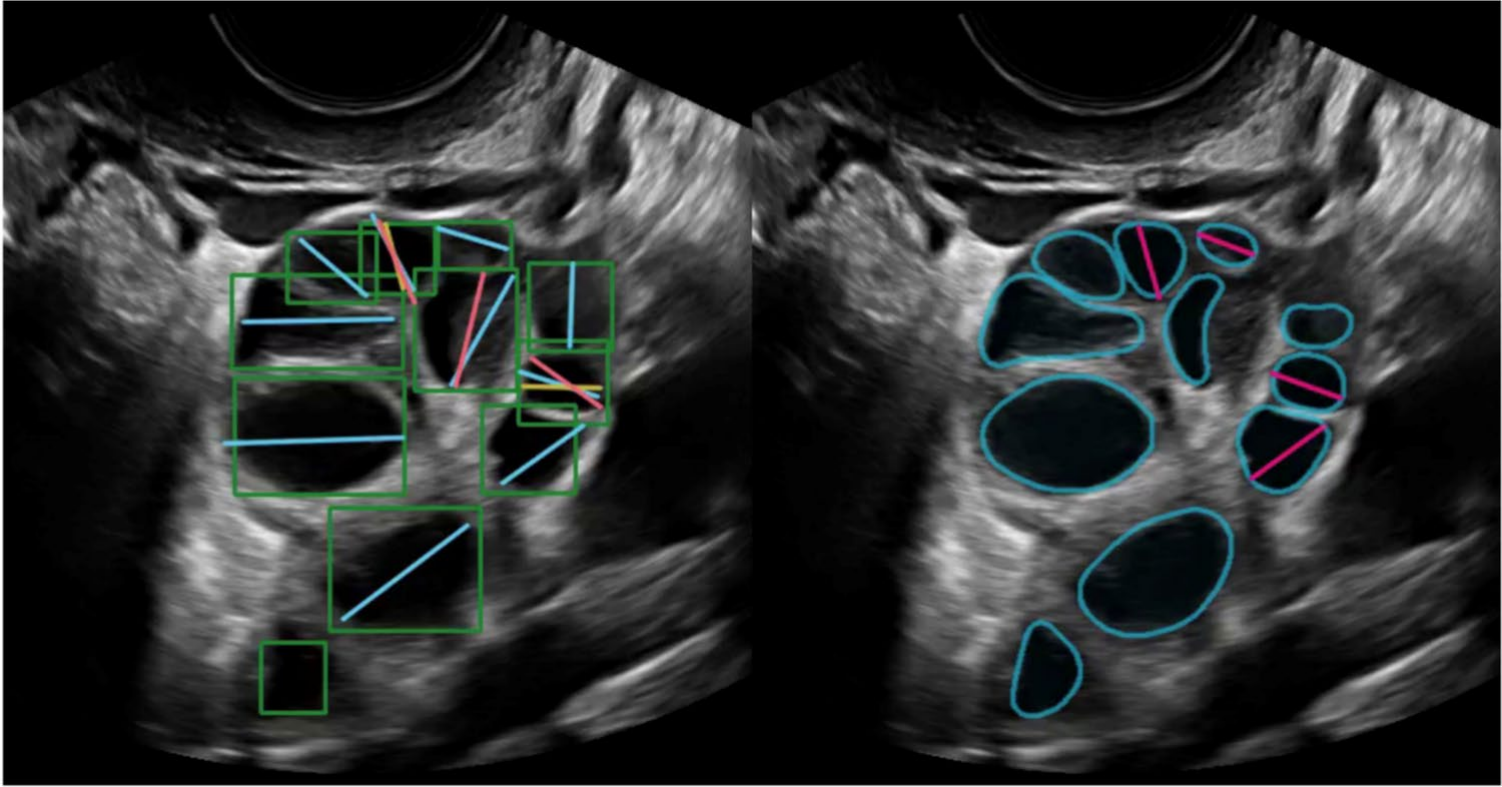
Scan from transvaginal ultrasound, B-mode (brightness mode)/2D Ultrasound. Left: example annotations from three independent experts (blue, yellow, red lines) and their consensus (green boxes). Consensus annotations are bounding boxes around follicles in each frame of the scan. Right, fully automatic outlines and their maximal diameters. Note that line annotations are only made on the frame where the follicle cross-section is judged maximal (experts tend to group these to a few distinct frames)
